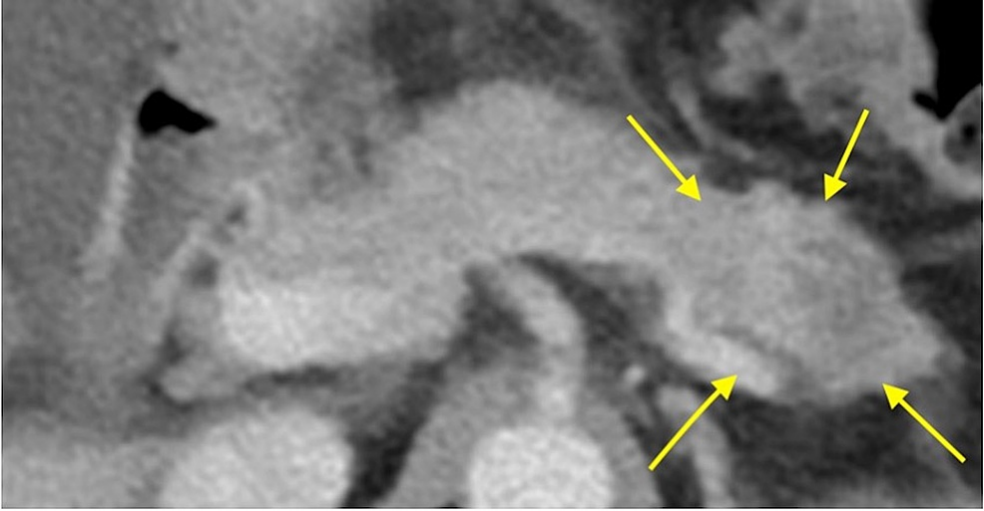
Computed tomography of the pancreas The tumor (yellow arrows) is located in the tail of the pancreas.
